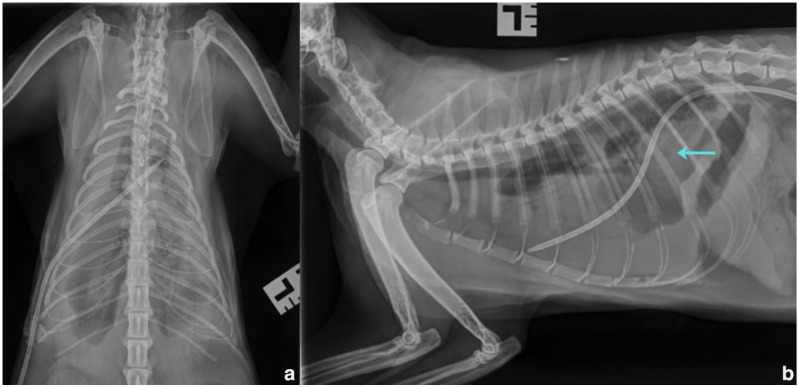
(a) Dorsoventral and (b) left lateral thoracic radiographs after chest drain placement. Soft tissue opacity in the caudal mediastinum (blue arrow) and bilateral pleural effusion evident
